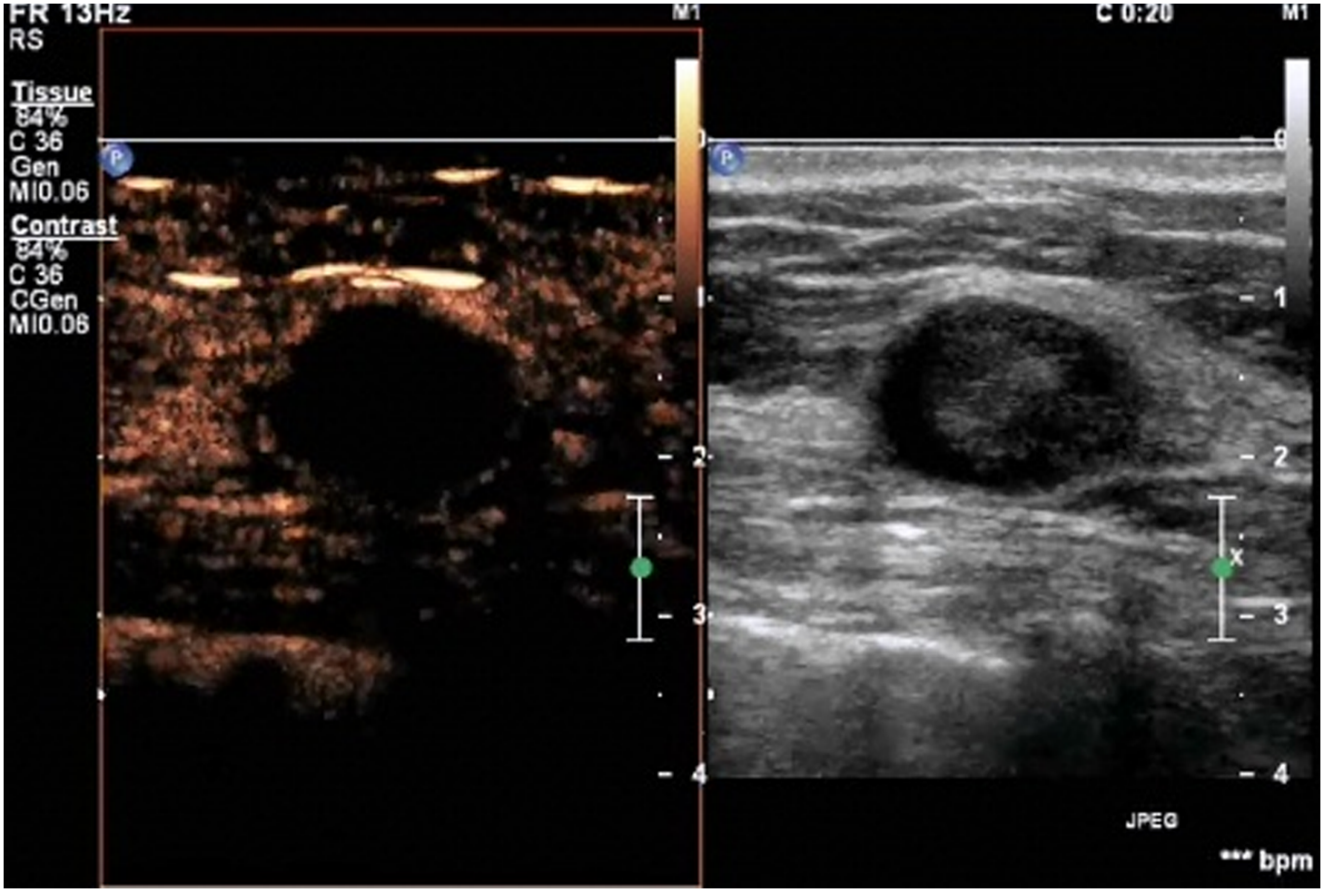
A lesion with a score of 1 by breast contrast-enhanced ultrasound. There is no enhancement in the lesion, with a clear borderline separating the lesion from the surrounding tissue.
